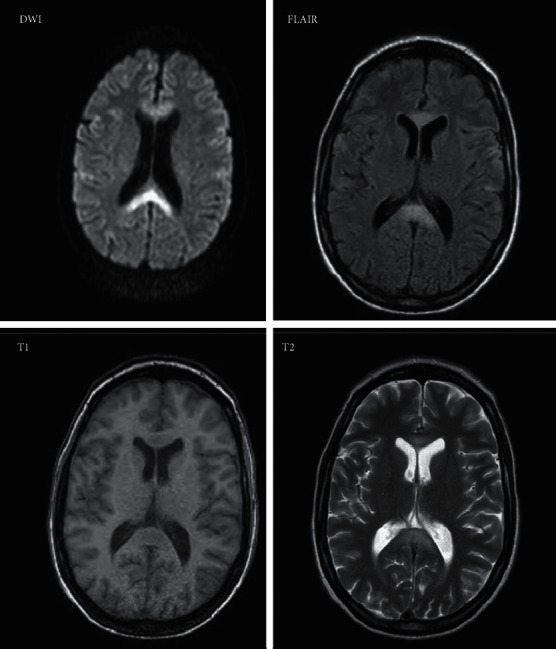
An MRI of the brain with a diffusion-weighted imaging (DWI) sequence showing restricted diffusion in the genu and splenium of the corpus callosum. A T1-weighted sequence shows hypointensity in the genu and splenium with corresponding hyperintensity on T2-weighted and T2-weighted fluid-attenuated inversion recovery (T2-FLAIR) images T2 and FLAIR. There is no enhancement noted with gadolinium (not shown).
